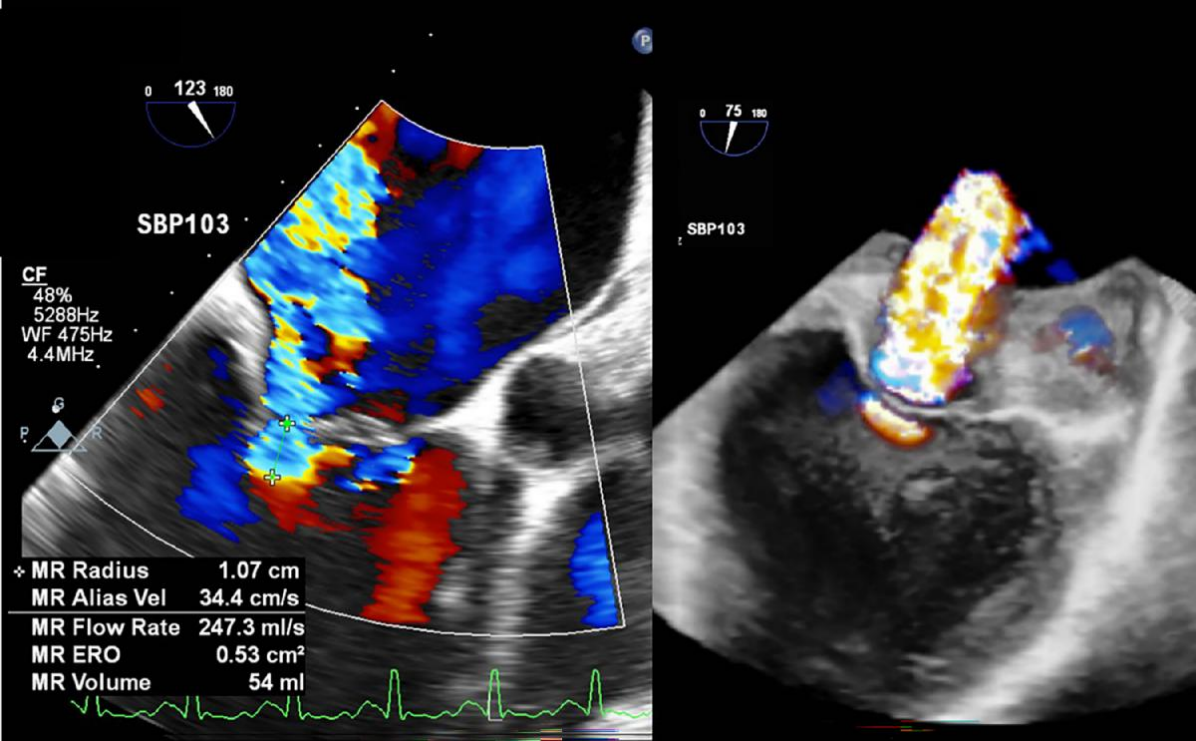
Transoesophageal echocardiogram (TEE) image for mitral regurgitation (MR). The TEE evaluation revealed MR (Carpentier type IIIB + I) with 2D effective regurgitant orifice (ERO) 0.52 cm^2^, 3D ERO 2.26 cm^2^, regurgitant volume 54 mL, and pulmonary vein reversal. The mitral valve (MV) structure was suitable for transcatheter edge-to-edge repair (MV area, 4.36 cm^2^; trans-MV mean pressure, 3.3 mmHg).
